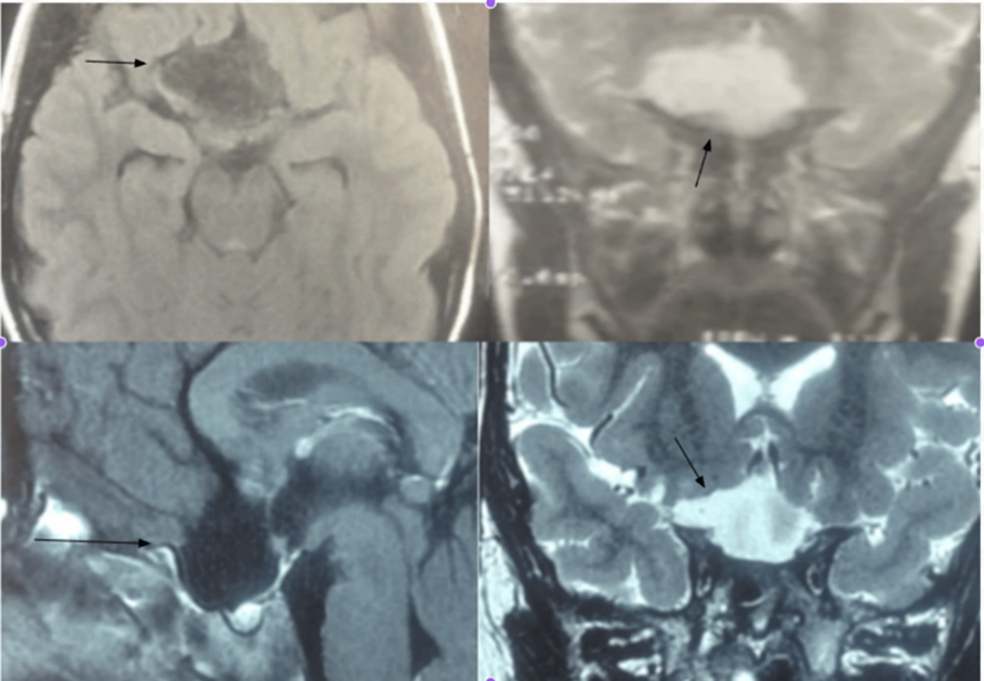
Cranial MRI MRI axial T1-weighted showing hypointense lesions in the parasellar region (top left image). MRI coronal T2-weighted showing hyperintense lesion in the parasellar region (top right image). MRI sagittal T1-weighted with contrast showing the lesion resection with preservation of the pituitary stalk (bottom left image). The resection can also be seen in the MRI coronal T2-weighted (bottom right image).
